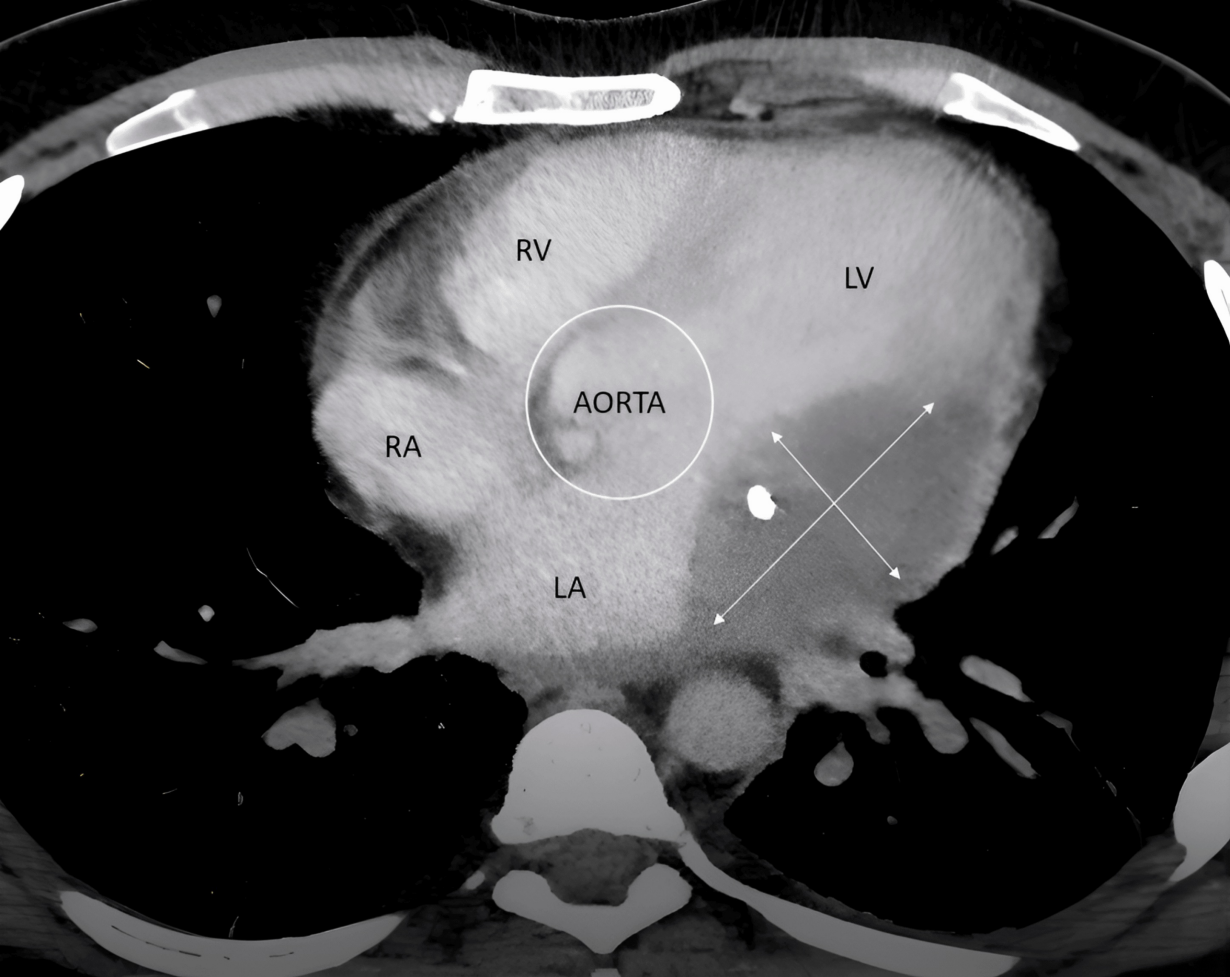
Cardiac CT suggestive of abscess in the left atrioventricular groove RA: right atrium; RV: right ventricle; LA: left atrium; LV: left ventricle
